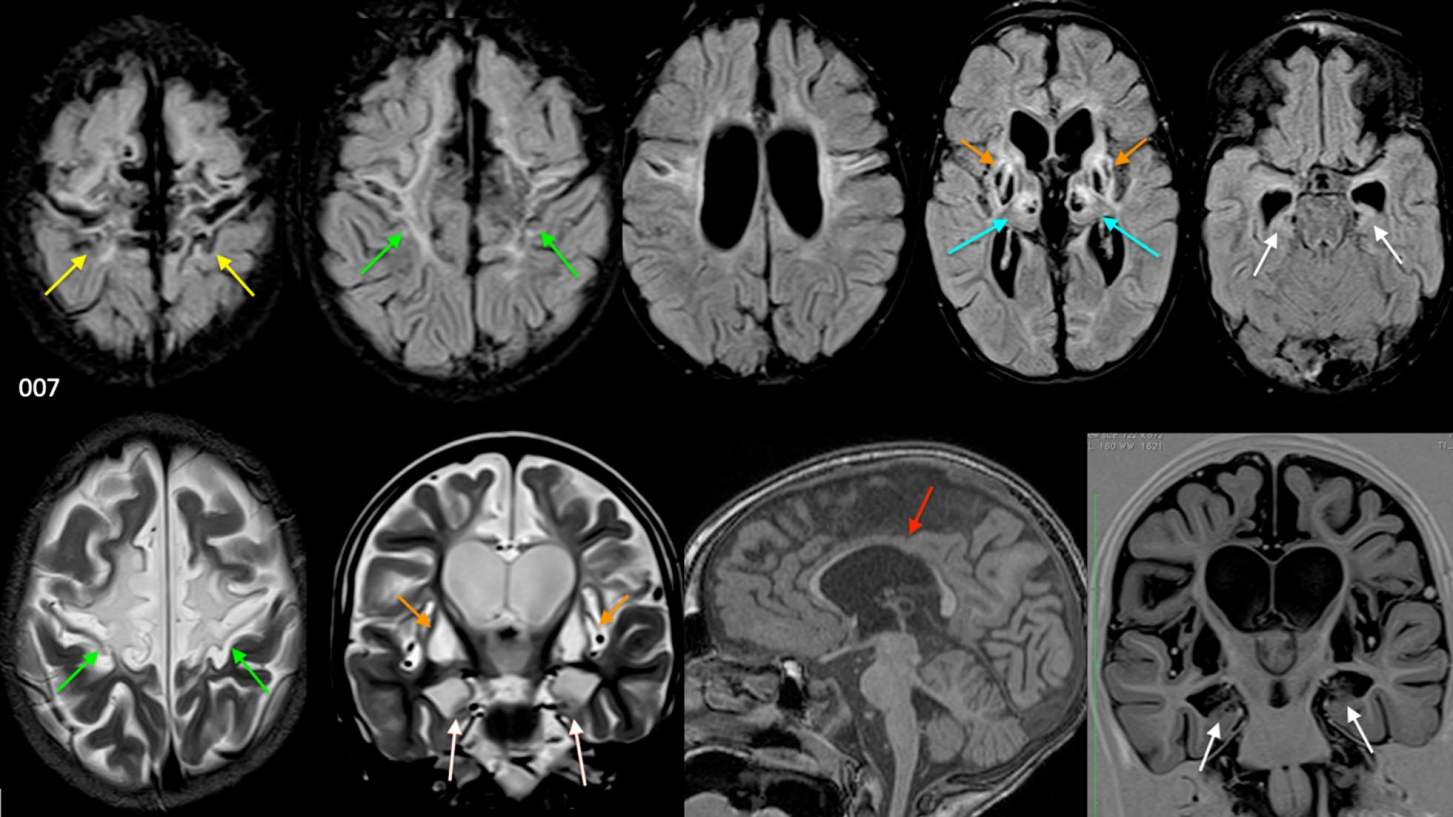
Collage of MR images demonstrating the key features of the massive paramedian injury pattern. Top row = axial FLAIR and bottom row (from left to right) axial T2-weighted, coronal T2-weighted, Sagittal T1-weighted and coronal inversion recovery sequence images. These demonstrate the perirolandic injury (yellow arrows), diamond-shaped expansion of the parasagittal cortex including the paracentral lobule (green arrows), hippocampal destruction (white arrows), putaminal necrosis (orange arrows) and thalamic (blue arrows) cavitation. The red arrow highlights the severe deafferentation thinning of the body and isthmus of corpus callosum
